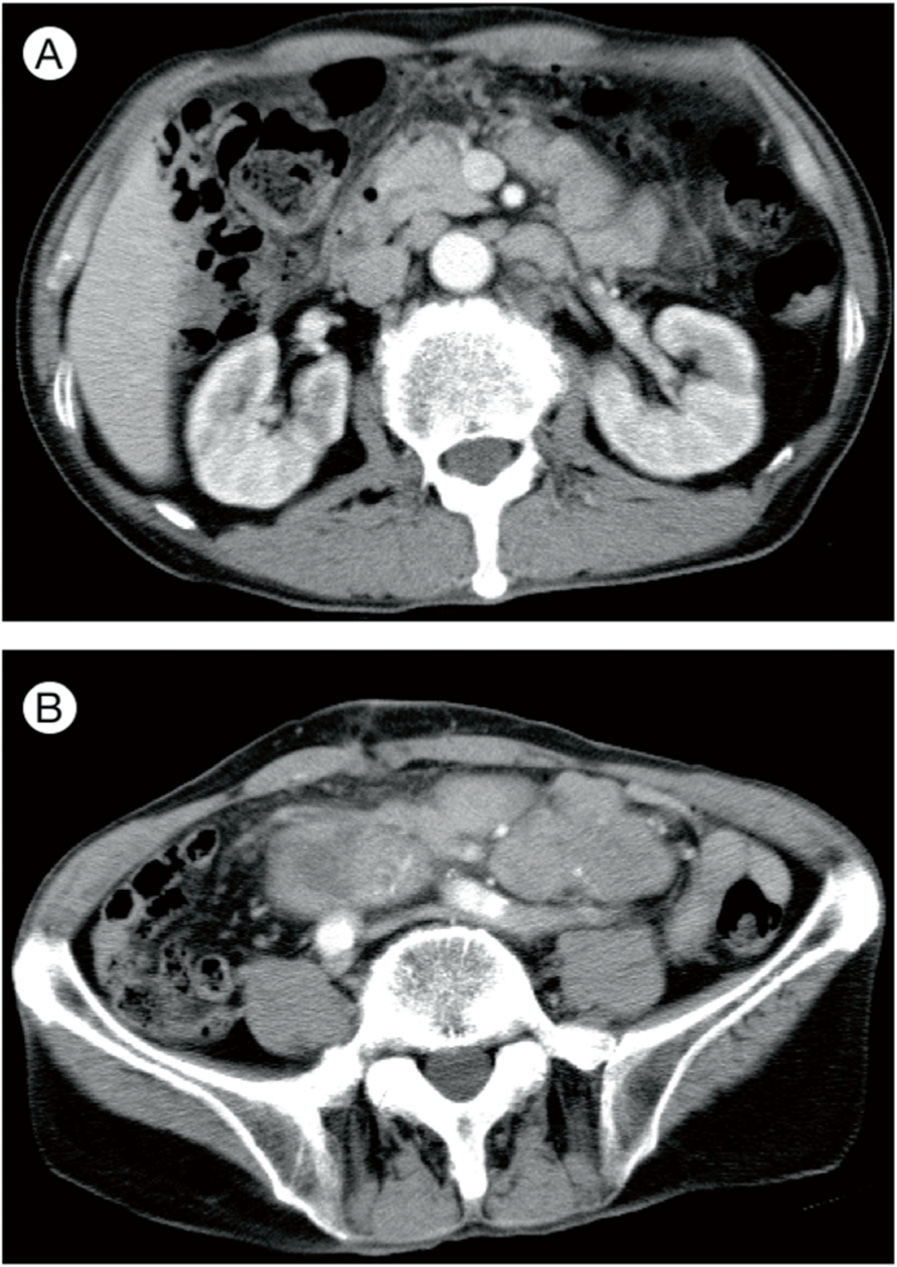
Radiological findings of the abdomen: Abdominal computed tomography scan revealed multiple lymph nodes were swollen in retroperitoneum (a) and mesentery (b)
